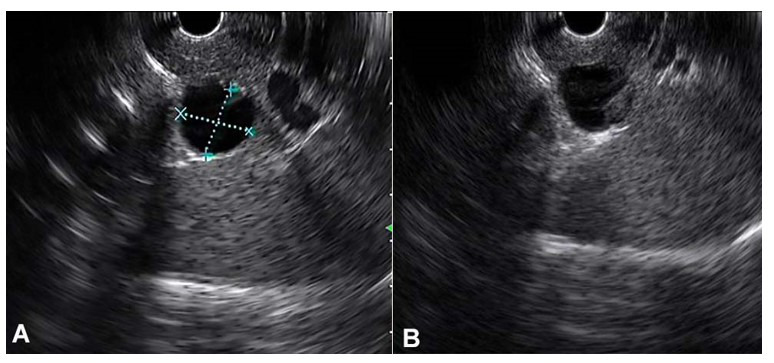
Endoscopic ultrasound with linear echoendoscope revealed a 1.6x1.5cm thinly septated cystic lesion at the pancreatic tail (A), with a suspicious 5mm mural nodule (B).
